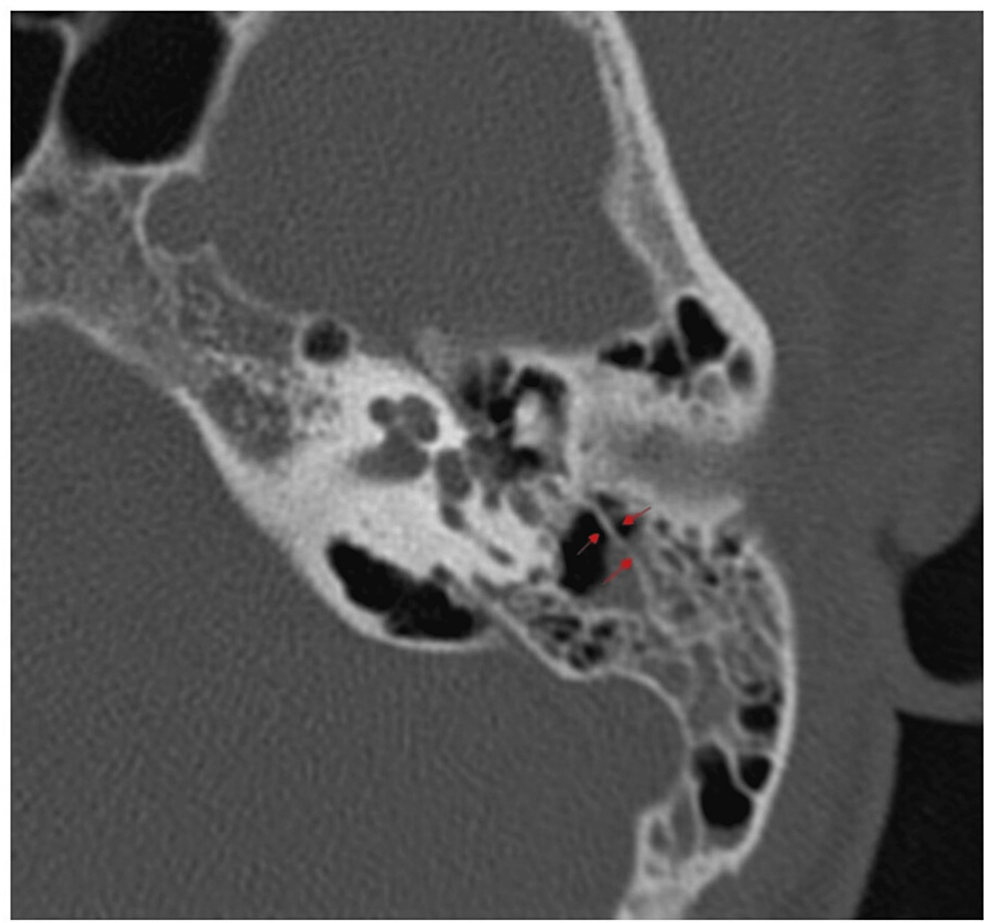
HRCT temporal bone: axial section with right ear showing the presence of Korner's septum (red arrows)
